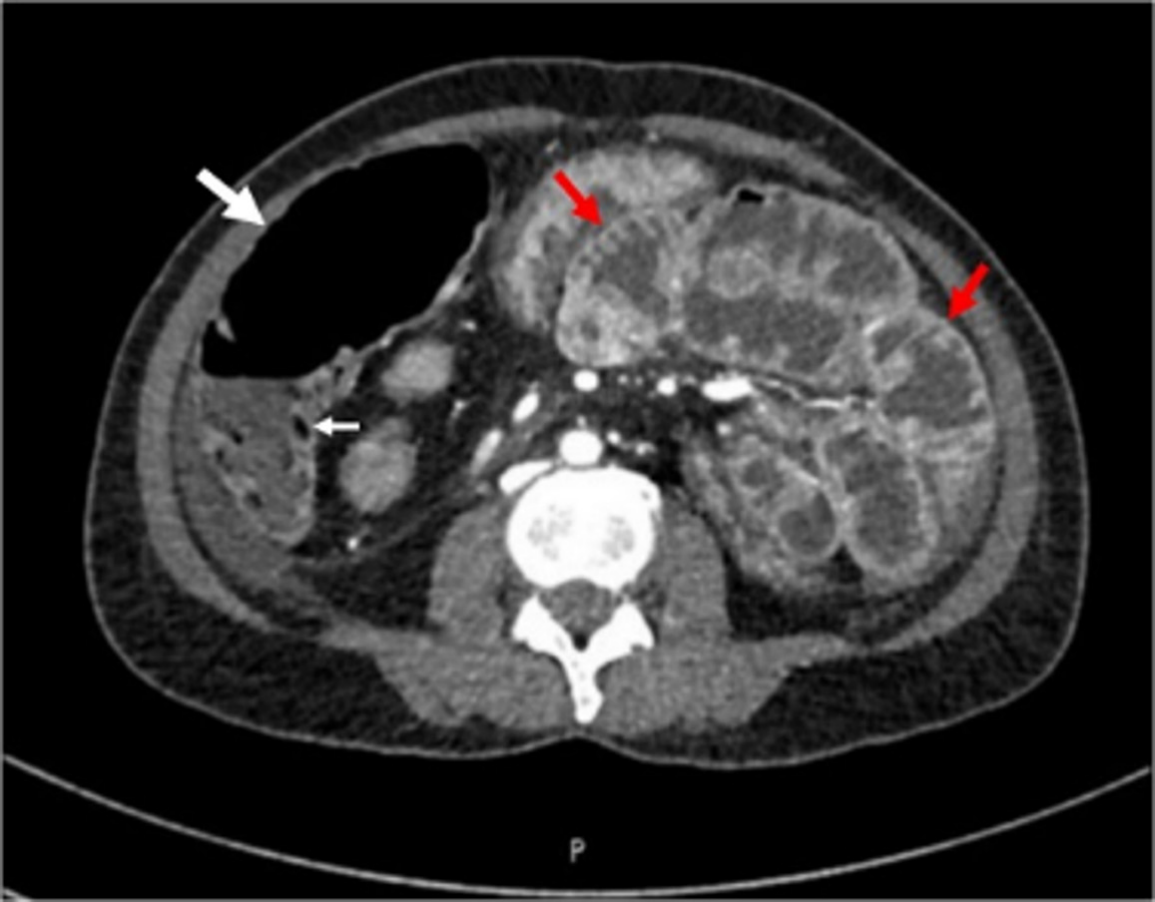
Computed tomography scan of the abdomen with contrast revealing mesenteric ischemia, with pneumatosis intestinalis (white arrows) and small bowel wall enhancement (red arrows).
